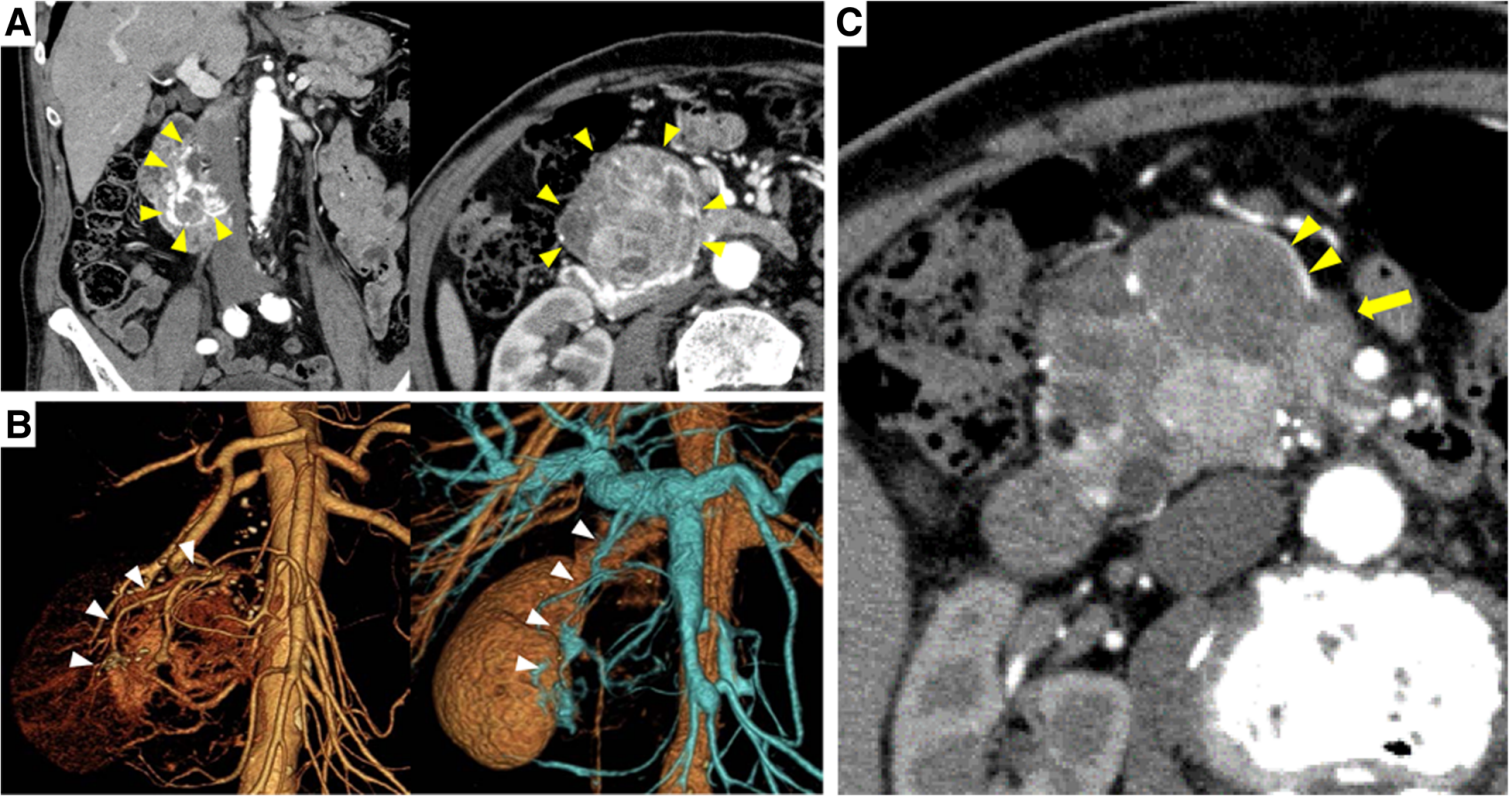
a Computed tomography (CT) of the serous cystic neoplasm (SCN) with hypervascularity in the pancreatic head and duodenum (arrow heads) during the late arterial phase. b Three-dimensional CT shows abnormal vasculature through the SCN in the arterial and venous phase (arrow heads). c The vein of the abnormal vasculature around the SCN was enhanced in the artery phase (arrow heads). The superior mesenteric vein was not enhanced (arrow)
